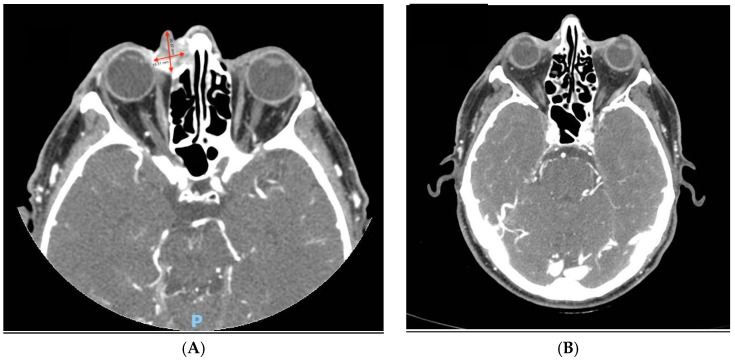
A 74-year-old male experienced impaired vision and ocular pain two weeks after the surgical excision of a cSCC at the medial canthus of his right eye. A head CT scan revealed a disease recurrence with evidence of a heteroplastic mass (12 × 20 × 21 mm, red arrows) with local invasion and bone involvement (A). To avoid a wide excision with exenteration of the orbit, treatment with cemiplimab was started. Following the first cycle, significant tumor regression was observed, resulting in a CR after 4 months (B). At the time of the analysis, the CR had been ongoing for more than one year and patient had regained complete vision.
